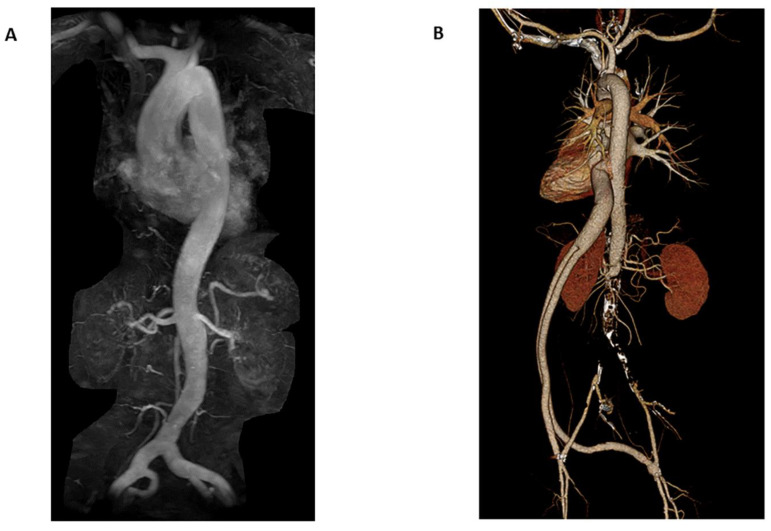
Coronal MIP MRA of the chest, abdomen, and pelvis (A) in a 66-year-old female for evaluation of possible aortic aneurysm demonstrates mild ectasia of the common iliac arteries bilaterally; however, no abdominal aortic aneurysm (AAA). 10 mL of Prohance contrast was administered. Coronal CTA volume rendered images (B) in a 70-year-old male with history of aortobifemoral bypass for suspected dissection demonstrates chronic occlusion of the infrarenal abdominal aorta and patent bypass. No dissection. 70 mL of Isovue contrast was administered with CTDIvol 19.2 mGy.
